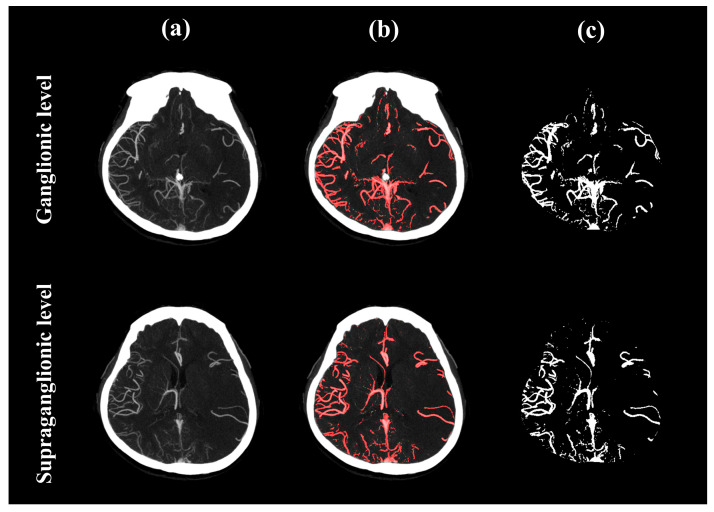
Example images of vessel segmentation. It includes (a) original images, (b) an overlay of the original images and the segmented vessel using the proposed method, and (c) binary images of segmented vessel. The images were obtained from a 69-year-old female patient with left hemisphere occlusion. CTA Menon score = 2.
